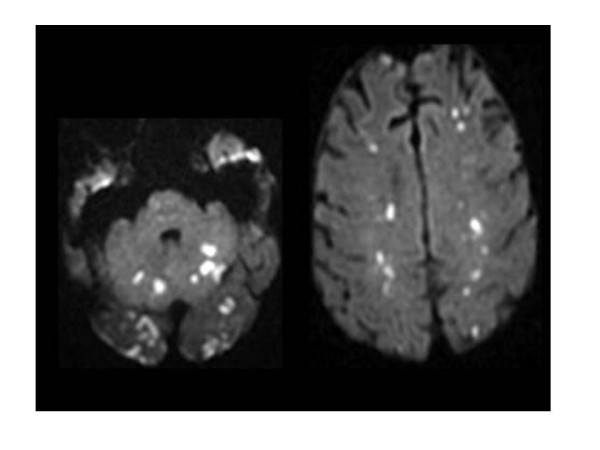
Diffusion-weighted magnetic resonance imaging. Acute hyperintense ischemic strokes in both hemispheres and in vertebro-basilar territories in the same patient (Reprinted from reference 12 with permission).
